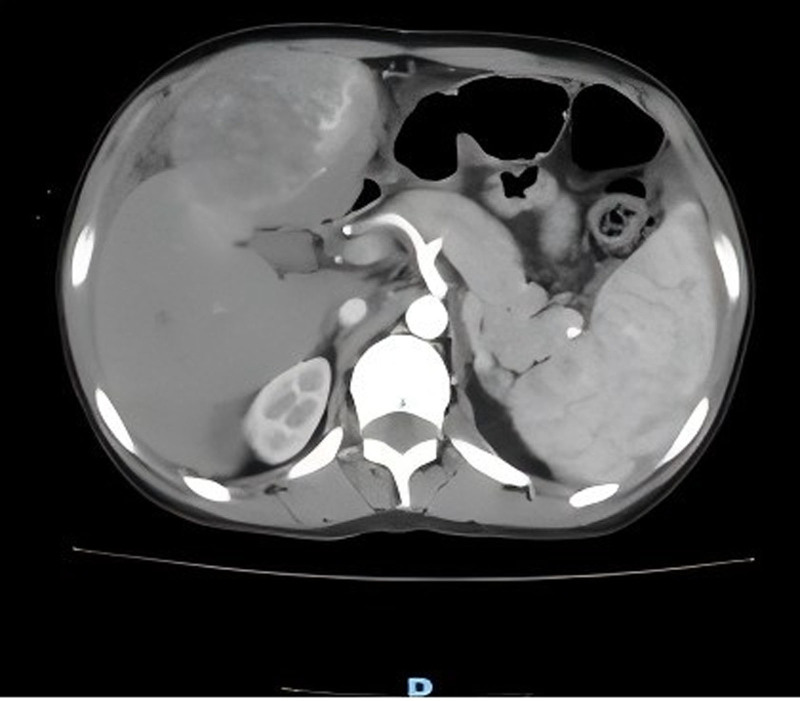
Abdominal enhanced CT: malignant mass in left medial lobe of liver (HCC possible), hemoperitoneum. CT = computed tomography, HCC = hepatocellular carcinoma.
